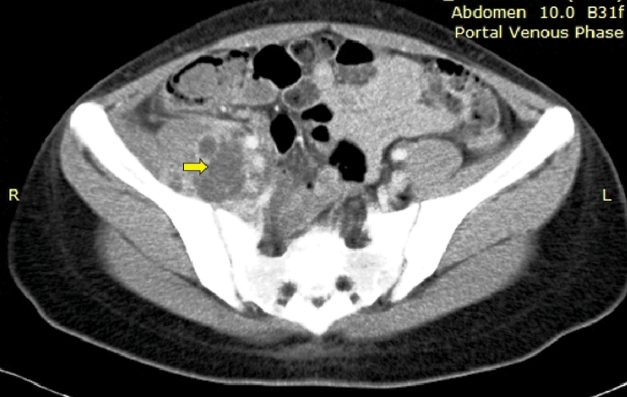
Computed tomography (CT) of the abdomen and pelvis shows multiloculated rim-enhancing collections at the right iliopsoas muscle
